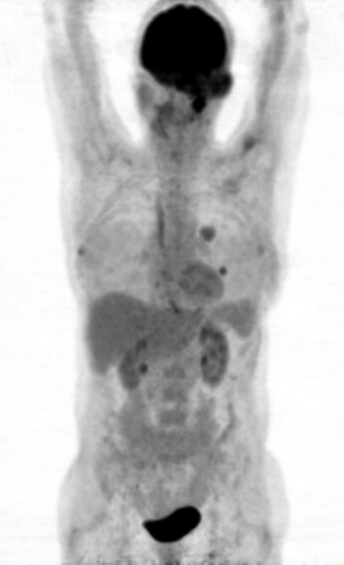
Maximal intensity projection visualisation of PET/CT demonstrating the intracranial meningioma and its pulmonary metastases.
